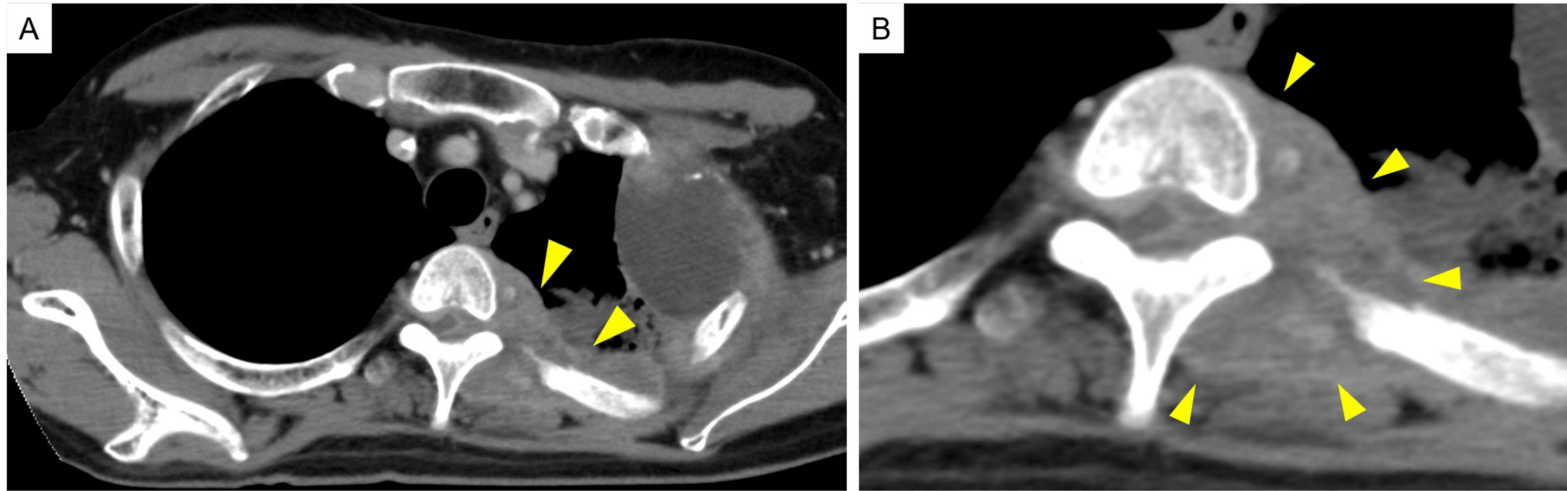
Image findings of metastatic tumors extended into the vertebral canal. Contrast-enhanced CT showing the metastatic third rib tumor extending into the vertebral canal through the intervertebral foramen between the second and third thoracic vertebrae (arrow head) (A). The magnification imaging of Fig. 5A shows the tumor mass compression of the spinal cord (arrow head) (B).
